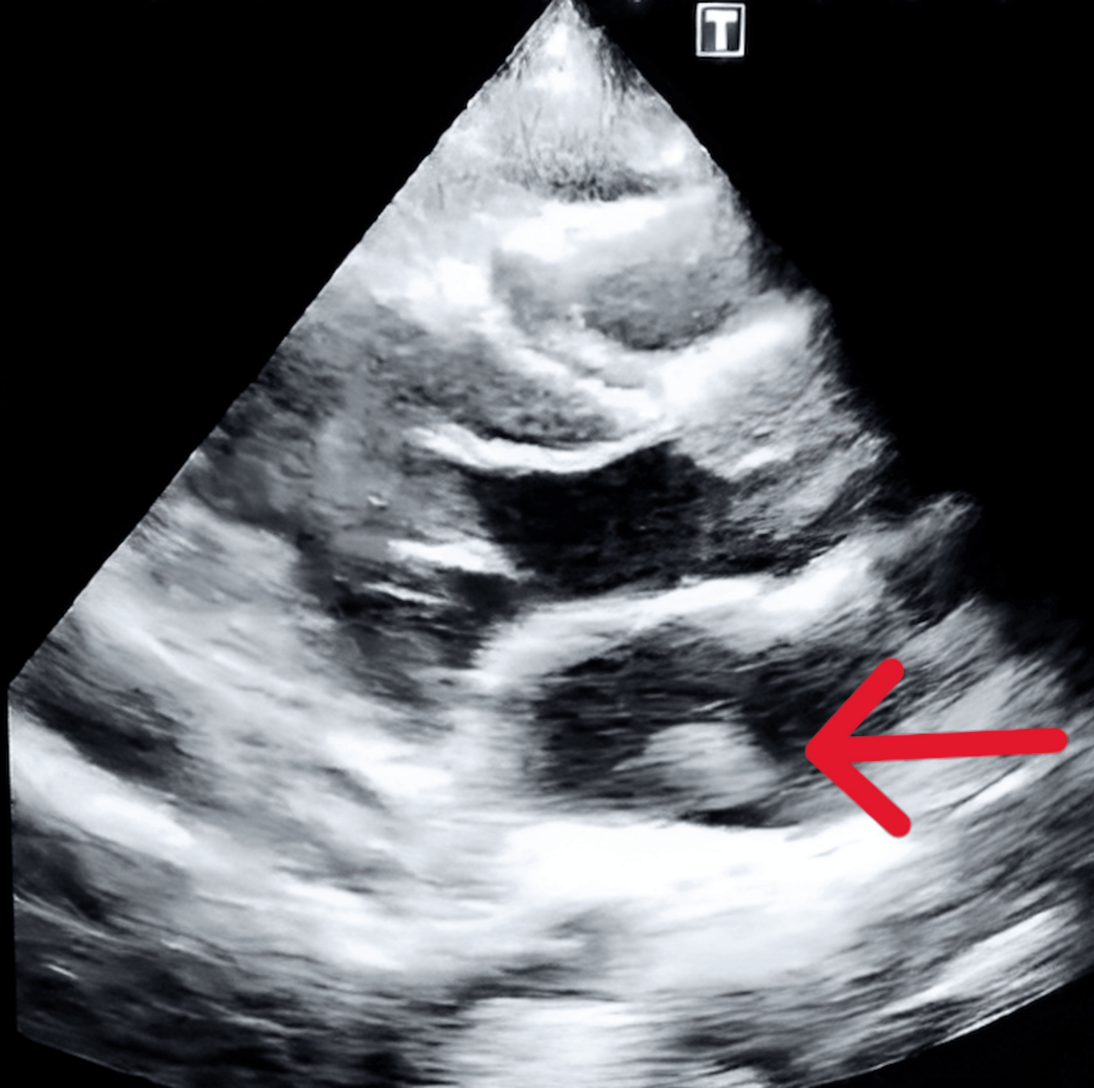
Echocardiogram showing a mobile oscillating mass measuring 11 x 15 mm in the left atrium
